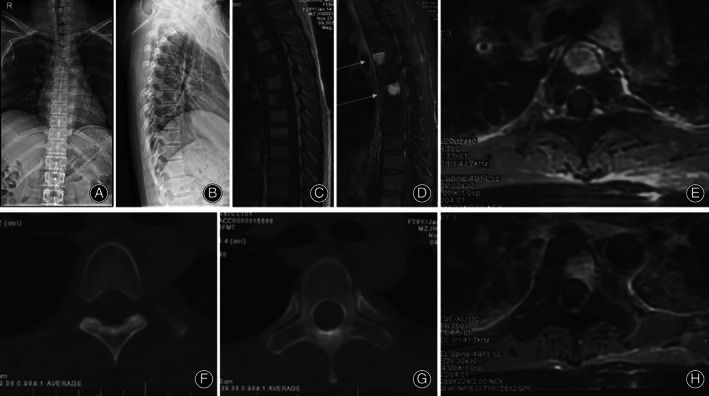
(A, B) Preoperative frontal and lateral radiograph X‐ray. (C–H) Preoperative MRI T1, T2 weighted images showing abnormal signal in thoracic 4–6 vertebral body. Preoperative CT showing slight reduction in vertebral density.
